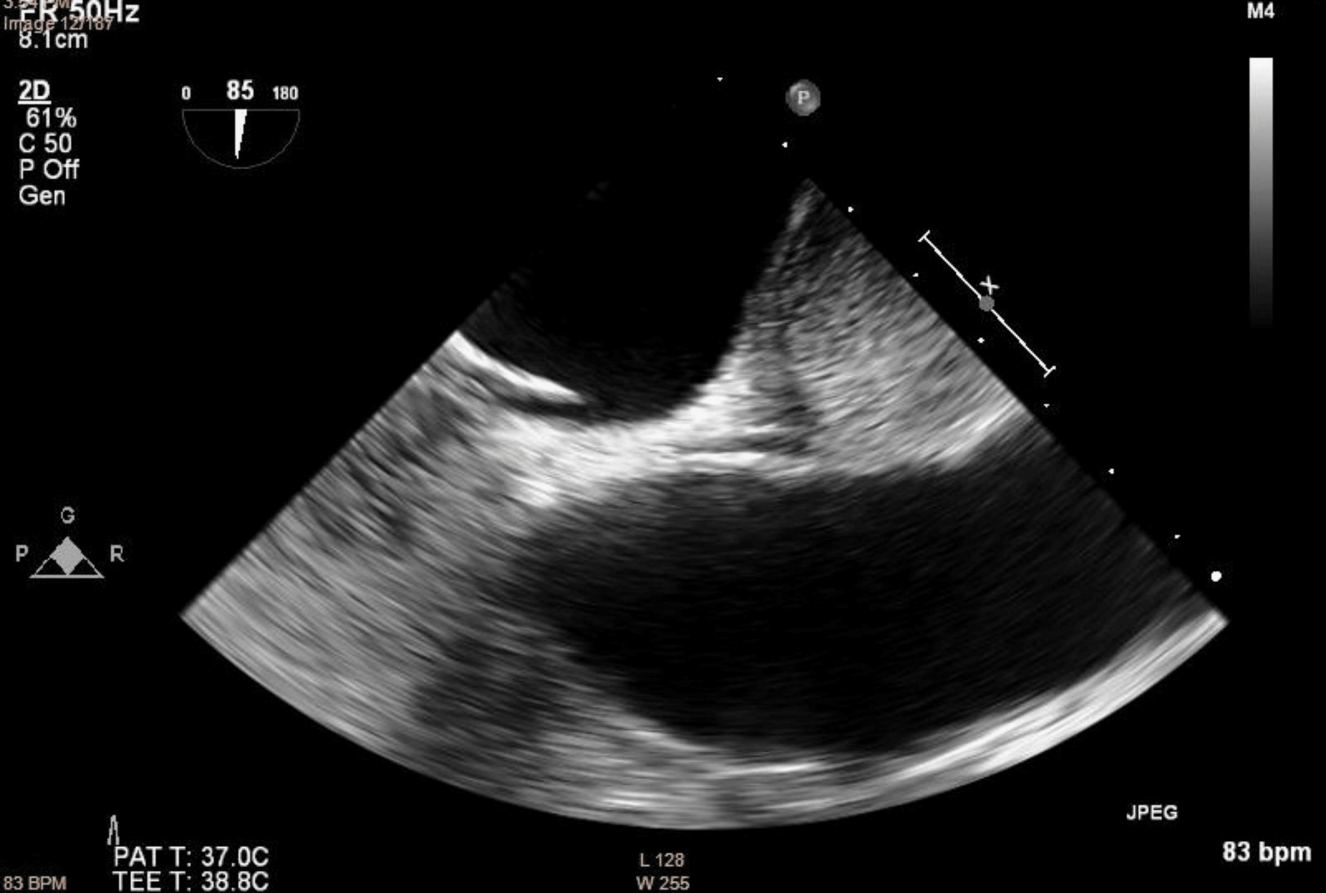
Transesophageal echocardiography of interatrial septum during bubble study: no signs of passing bubbles through the interatrial septum
